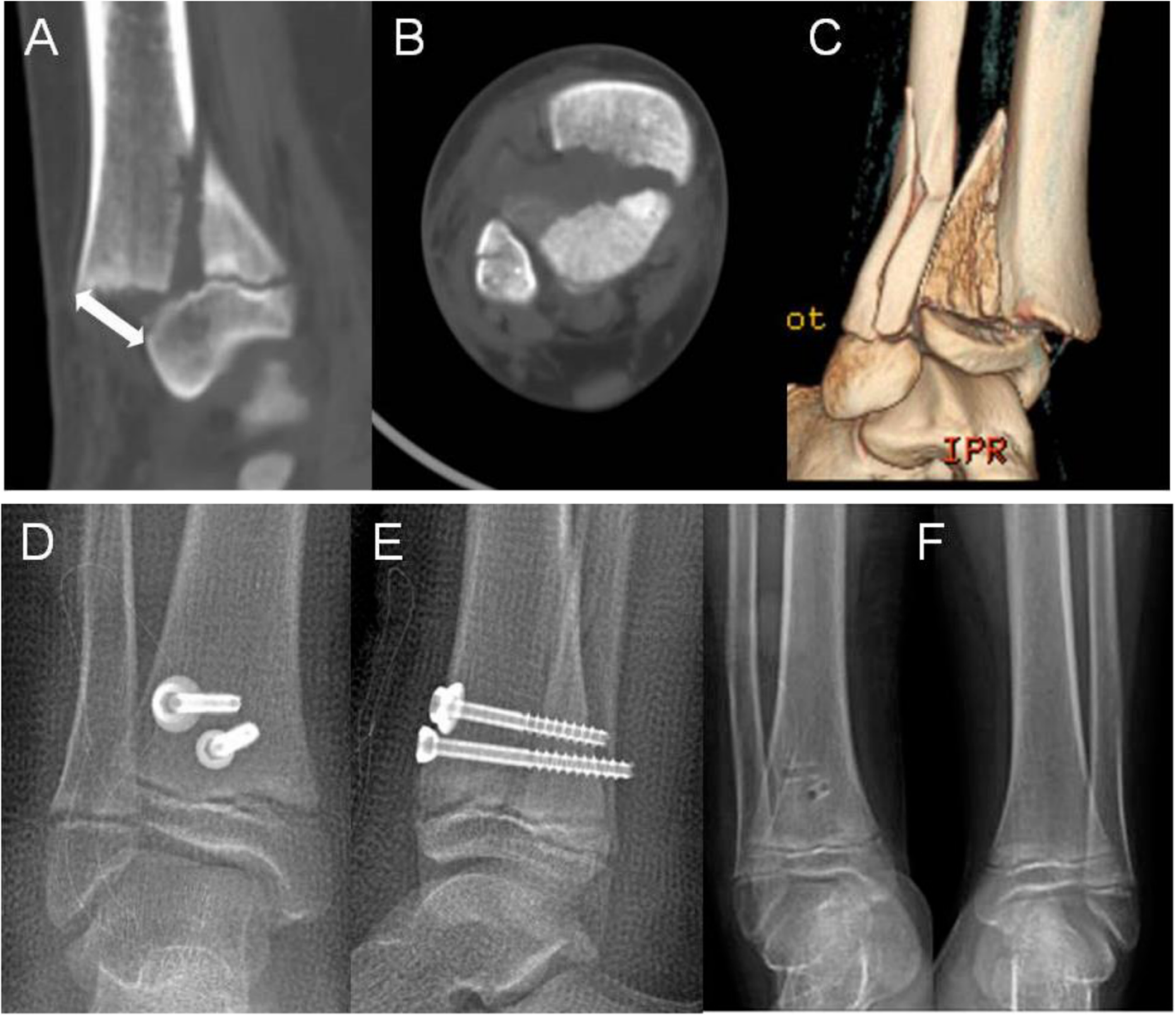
A 12-year-old boy. a–c, Sagittal, axial and 3D view demonstrated 15 mm displacement of the fracture (white double arrow ). d, e AP and lateral radiographs demonstrated the anatomical reduction immediately post operatively. f AP radiograph of bilateral ankle joints taken at 9-month follow-up showed symmetrically open physis as compared with the opposite side
